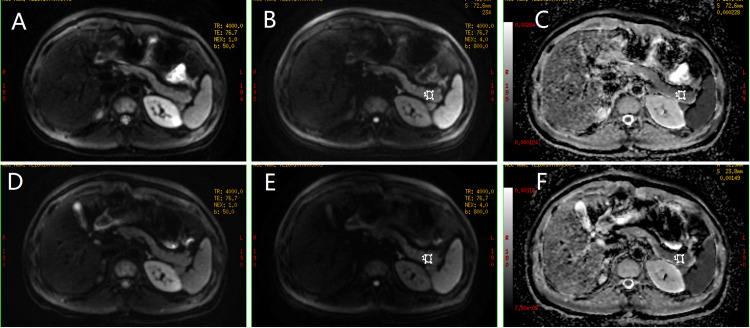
Representative images were acquired from a volunteer with test-retest DWIs. DWI images with b-value of 50/800 s/mm^2^ (A, B) and corresponding ADC map of first DWI (C); (D-F) images and the ADC map of the retest DWI. DWI - diffusion-weighted imaging ADC - apparent diffusion coefficient
